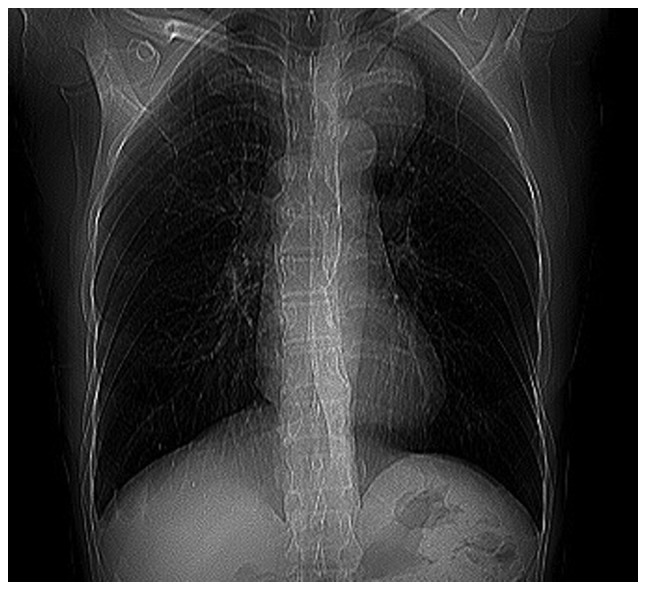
Posteroanterior chest roentgenogram demonstrating a left superior lung field mass protruding from the mediastinum.
